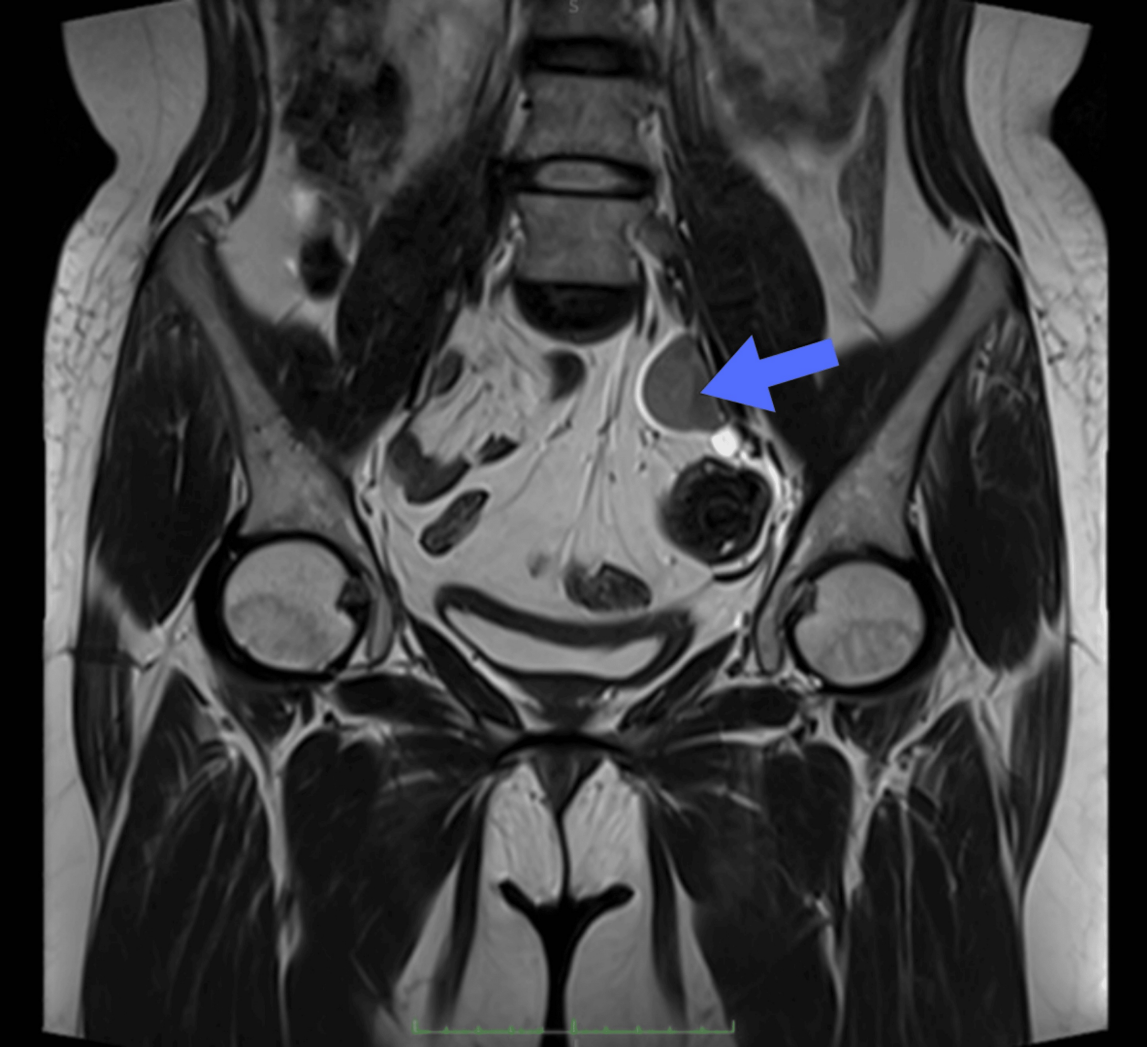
MRI pelvis scan showing well-defined ovoid tissue in the left iliac region (blue arrow)
